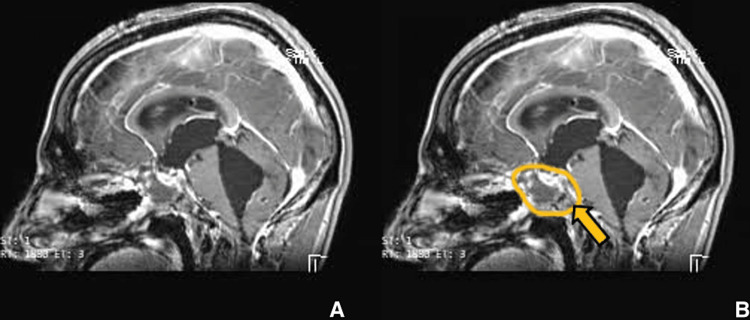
Postoperative imaging for patient 1. Contrast-enhanced T1-weighted sagittal image showing significant reduction in lesion size. (A) Without ROI and (B) with ROI. Post-surgical changes are shown, including interventional ventriculoperitoneal (VP) shunt.
